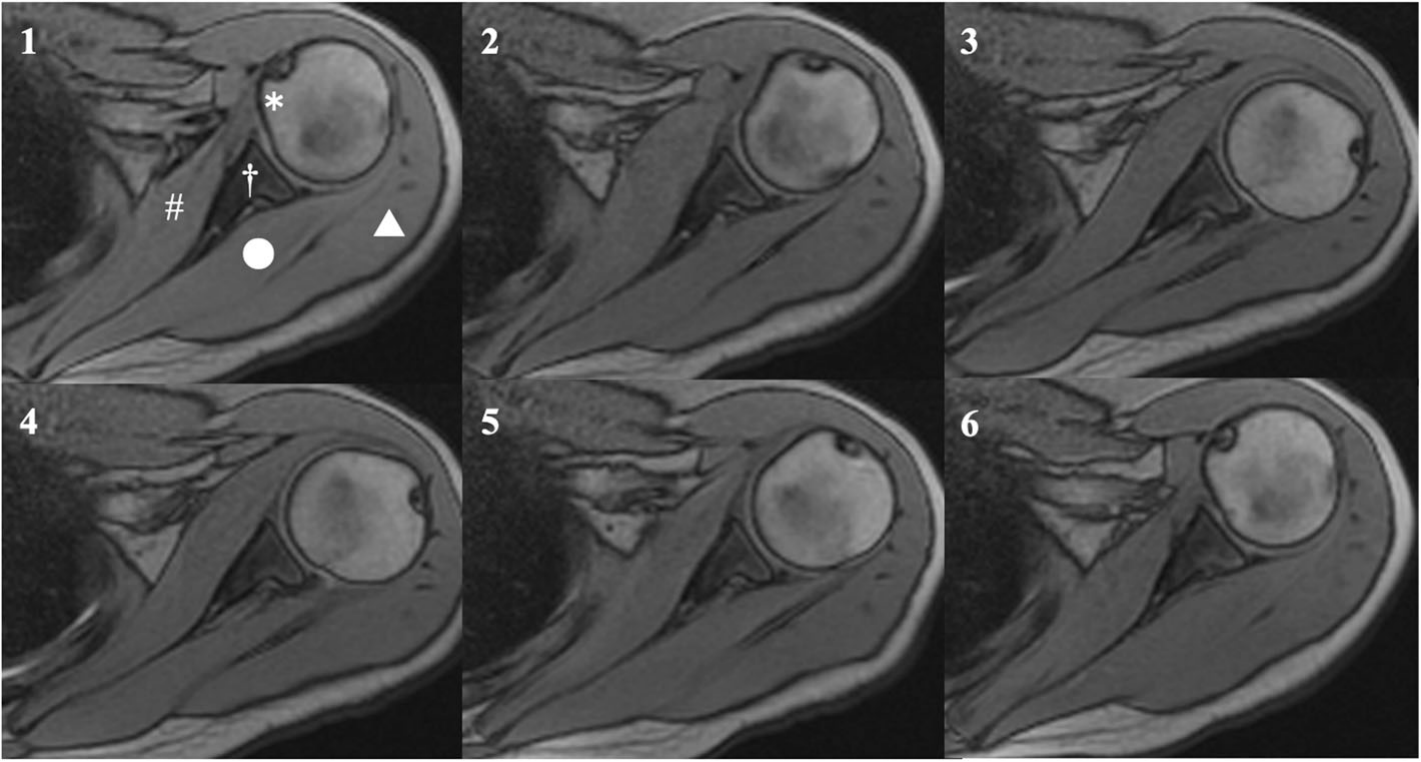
Motion of an asymptomatic volunteer. The left shoulder of a participant. The movement of the subscapularis and infraspinatus muscles of an asymptomatic shoulder was clearly visible using cine-MRI and no blurring was observed. 1, Starting position; 2, Motion in external rotation; 3, Point at the maximum external rotation; 4 and 5, Motion in internal rotation; 6, End position. *, The lesser tuberosity of the humeral head; #, The subscapularis; †, Glenoid; ○, The infraspinatus; △, The deltoid
